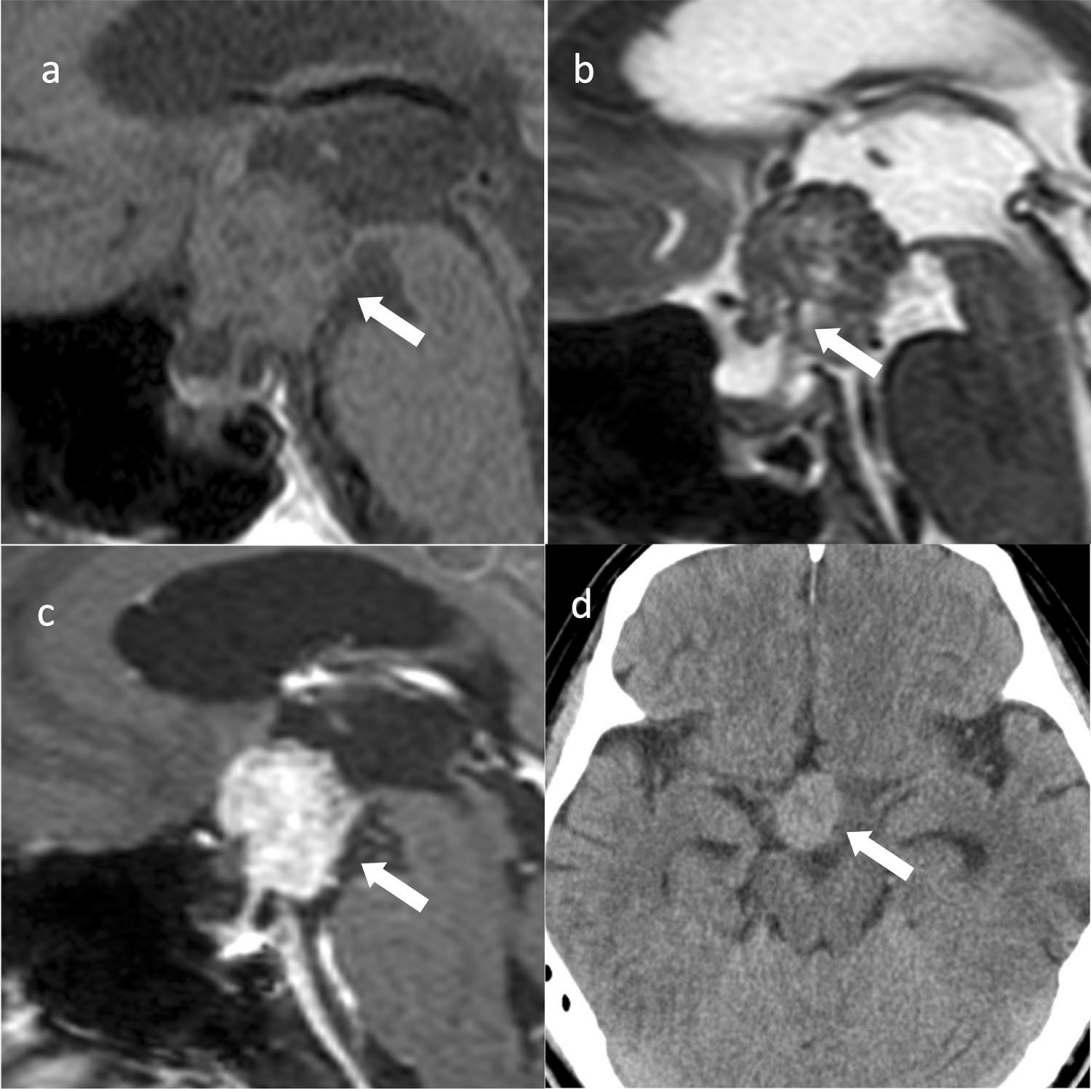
Papillary craniopharyngioma. An approximately 50-year-old male with eye floaters. a Sagittal T1WI shows a mass with isointensity compared to white matter from the suprasellar region into the third ventricle (arrow). b Sagittal T2WI shows some small cyst-like hyperintensity areas in a iso- to hypointense mass compared to white matter (arrow). A duct-like recess is observed at the base of the mass contiguous with the pituitary stalk (arrow). c Sagittal contrast-enhanced T1WI shows almost uniform and strong contrast enhancement (arrow). d Non-contrast computed tomography shows mild high density (arrow). The patient underwent surgery via nasal endoscopy, and the mass was confirmed as a papillary craniopharyngioma arising from the base of the third ventricle
